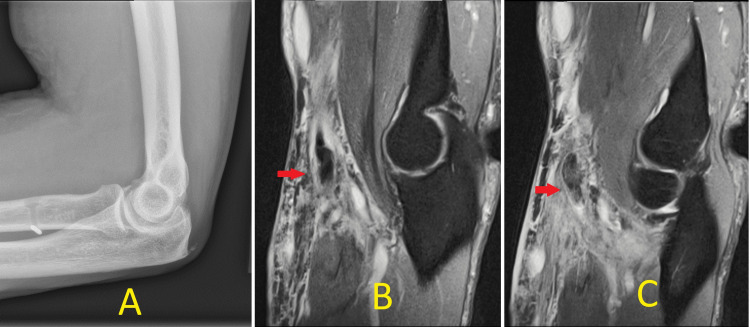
Imaging studies of the left elbow. The lateral radiograph (A) shows post-surgical changes with intact cortical button. MRI coronal T2-weighted slices (B, C) showing proximal retraction (arrow) of repaired distal biceps stump.
